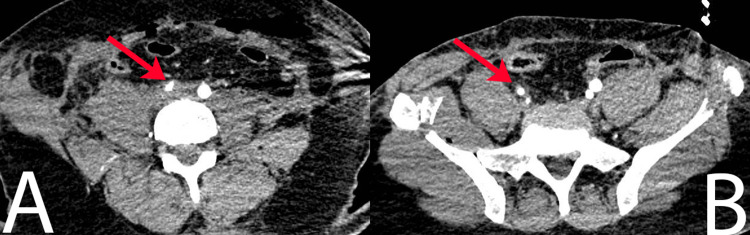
Axial CT images of the common iliac artery. (A) CT image demonstrating right common iliac artery near-total luminal occlusion caused by a thrombus. (B) CT image demonstrating attenuation of the right external and internal iliac arteries in comparison to the contralateral side. CT: computed tomography
